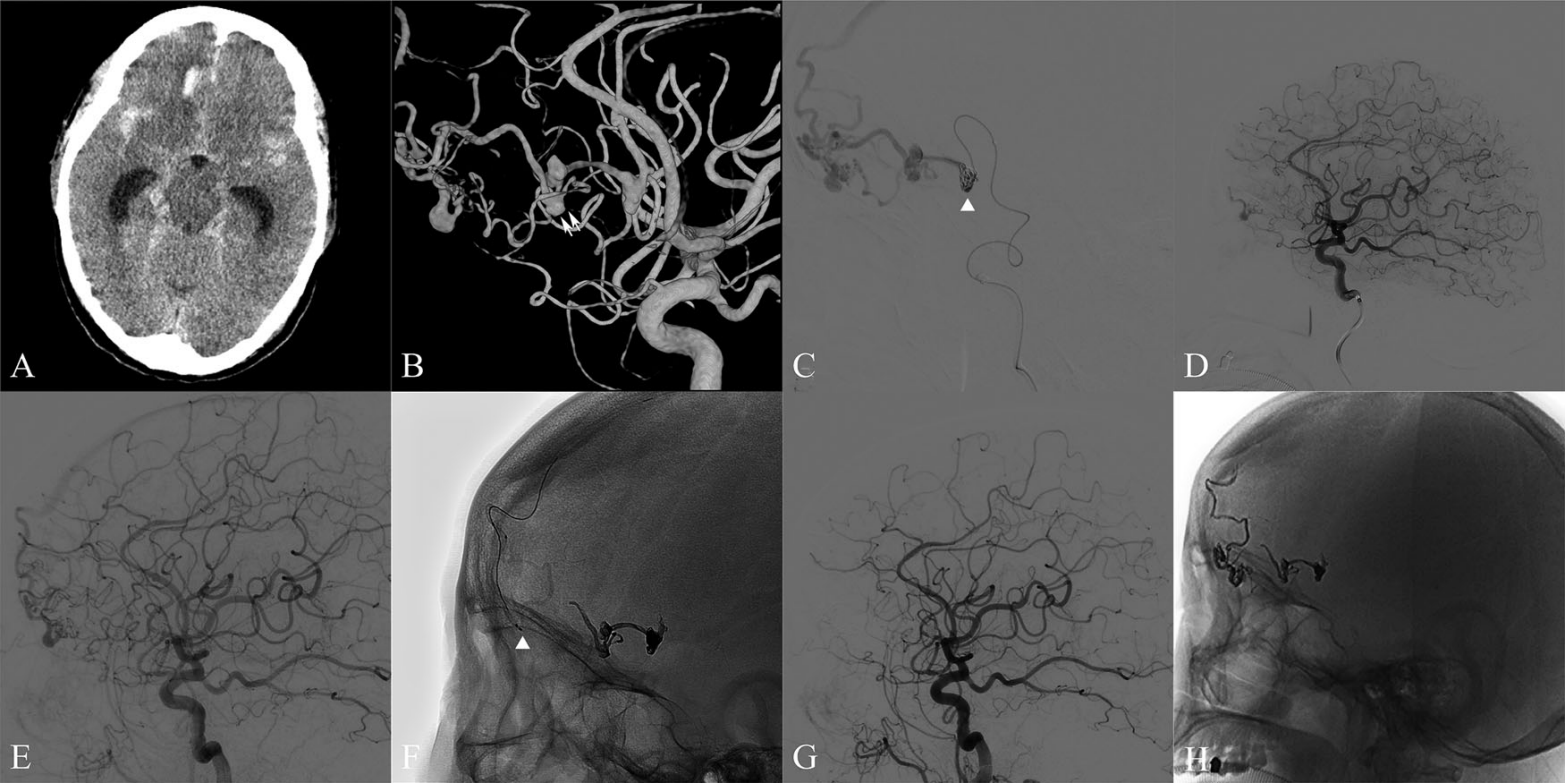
The patient was admitted to the emergency department with a sudden severe headache. Initial non‐contrast CT revealed extensive subarachnoid hemorrhage (A). Lateral right internal carotid angiogram with 3D reconstruction demonstrated a frontal base vascular malformation with flow‐related aneurysms on the orbitofrontal artery (B). Multiple tandem flow‐related aneurysms along the orbitofrontal artery were demonstrated by microcatheter angiography, with the largest one located at its origin (white arrow head) (C). Endovascular treatment of the aneurysms was performed using two coils and subsequent injection of 1.2 mL Onyx‐18. Post‐procedure angiogram confirmed complete aneurysm occlusion with decreased AVM flow (D). At planned second‐stage intervention 40 days later, a lateral right internal carotid angiogram revealed increased AVM flow with a partially obscured nidus (E). Non‐subtracted imaging demonstrated the Onyx cast from the initial procedure, and Dual Echelon‐10 microcatheters were navigated through the superior sagittal sinus to access the cortical veins (white arrow head) (F). Following deployment of two coils in the proximal draining vein, 1.8 mL Onyx‐18 was administered. Final lateral right internal carotid angiogram confirmed complete AVM obliteration (G). Non‐subtracted image showed the final Onyx cast after the second intervention (H).
